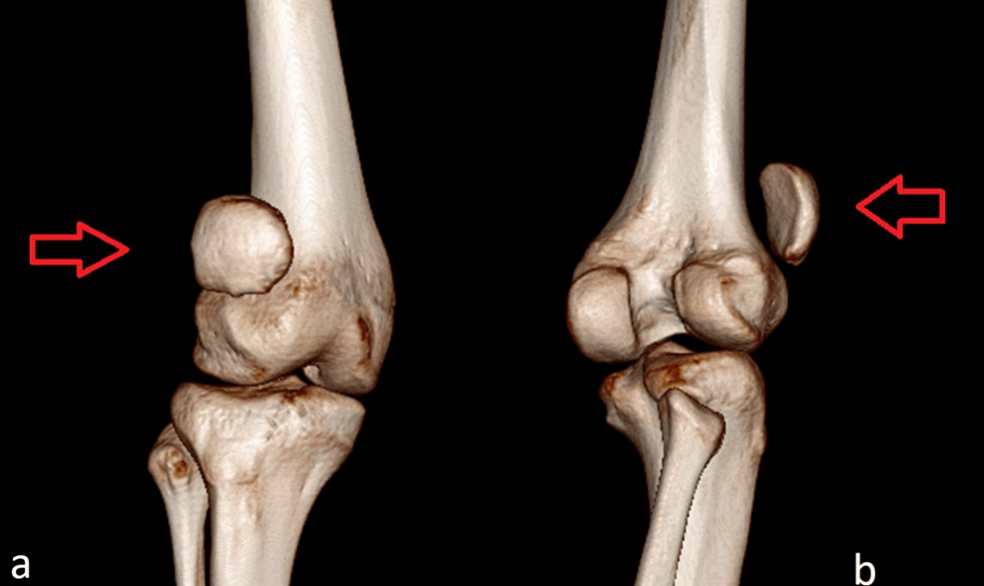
CT scan of the right knee with arrows showing superolateral displacement of the patella and posterolateral translation of the tibia with respect to the femur (a) Anterior view; (b) Posterior view
